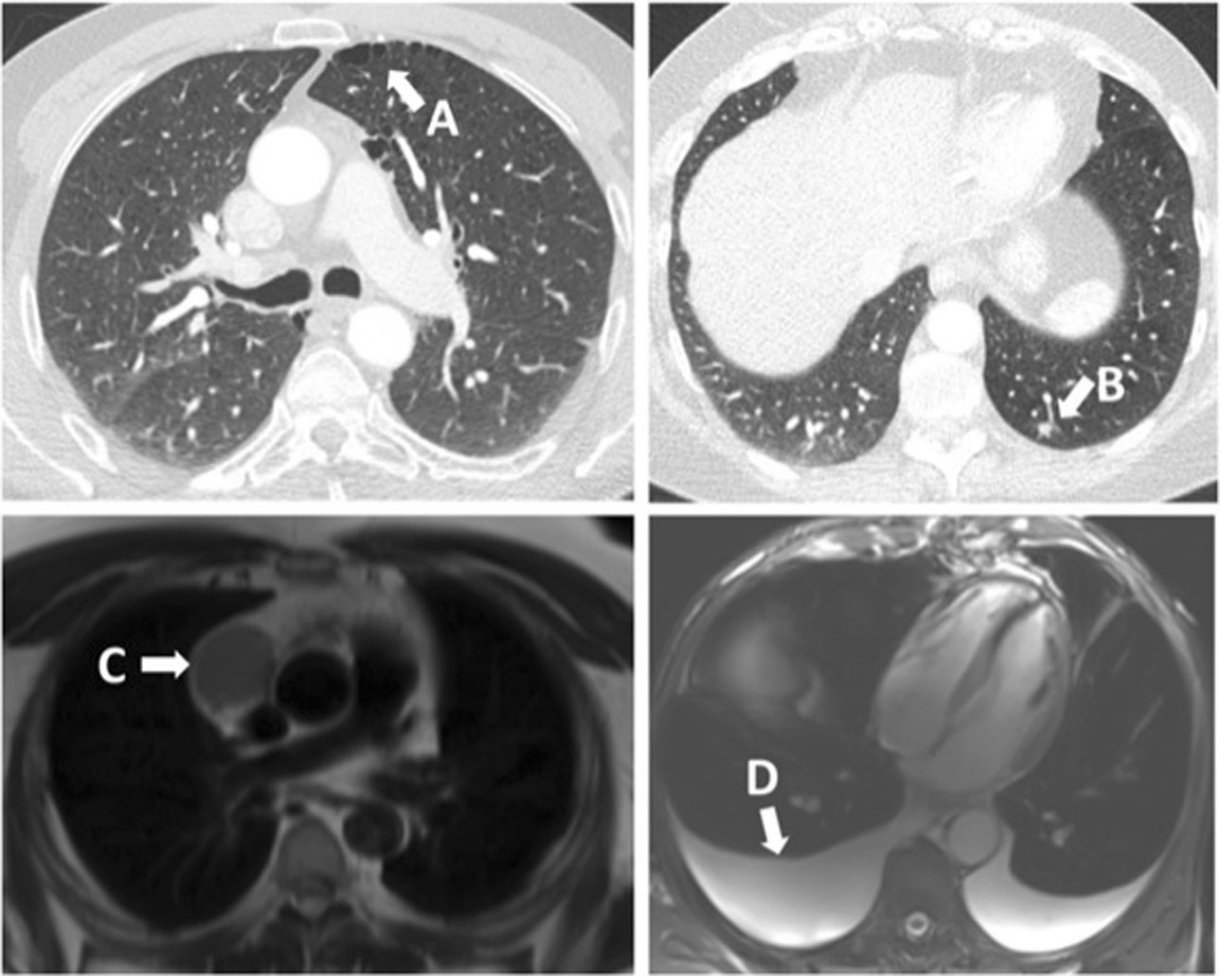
CT and MRI images of common INCF (A, paraseptal emphysema on CT; B, 7-mm pulmonary nodule on CT which requires follow-up imaging; C, mediastinal lymphadenopathy on MRI; D, pleural effusion on MRI).
